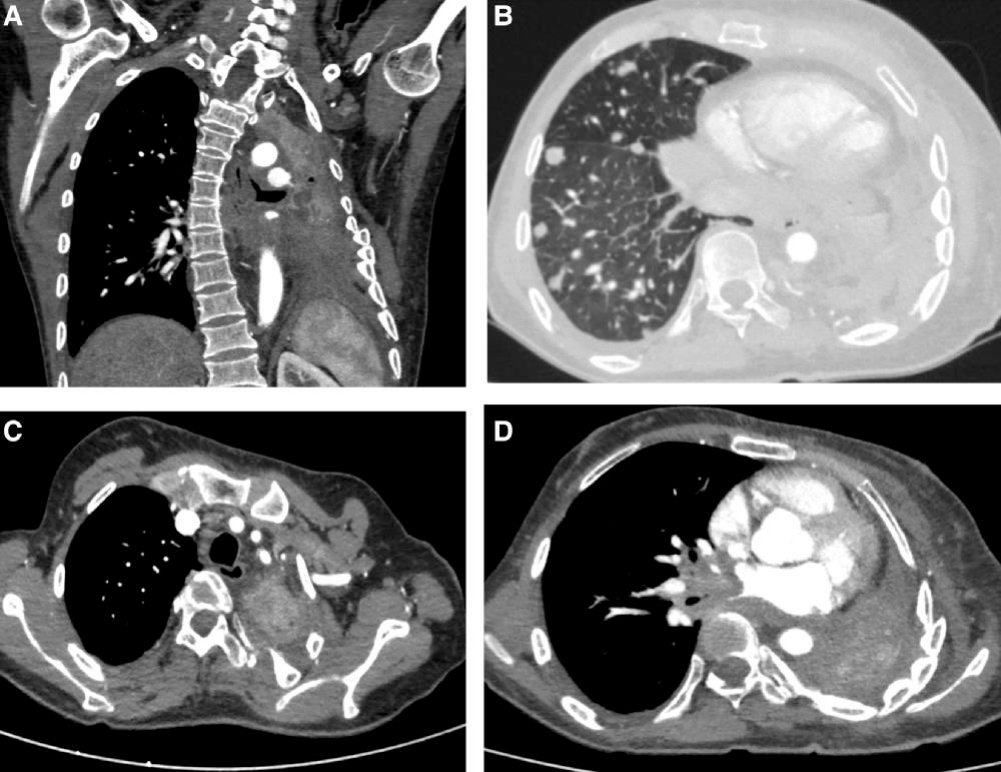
Contrast-enhanced computed tomography of the thorax revealed the collapse of the left lung with fibrotic changes (A), multiple nodules in the right side of the lung with mediastinal lymphadenopathy (precarinal, right upper, and lower paratracheal, with right hilar being largest at1.5 × 1.6 cm) (B), heterogeneously enhancing nodular lesions in the extrapleural space on the left side extending along the intercostal nerves in the ninth, 10th, 11th, and intercostal space on the left side, the largest measuring 9.6 × 9.5 mm (C and D).
